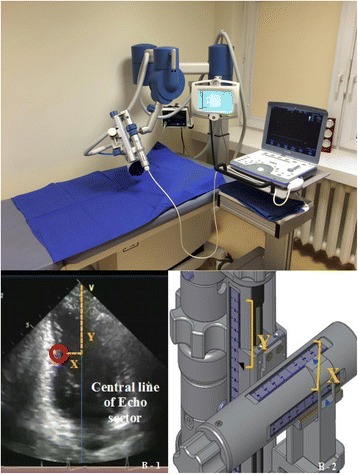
The methodology of cardiac shock wave therapy. a Shock wave generator system (Medispec, Germantown, MD, USA) and cardiac imaging system (Vivid i, GE Healthcare, Horten, Norway). b Shock wave focal zone alignment: Position of the sub-segment on the 2-dimensional image determined by X and Y coordinates (1). The shockwave applicator position is identically adjusted along X- and Y-axes corresponding to the X and Y coordinates of the ultrasound image (2)
